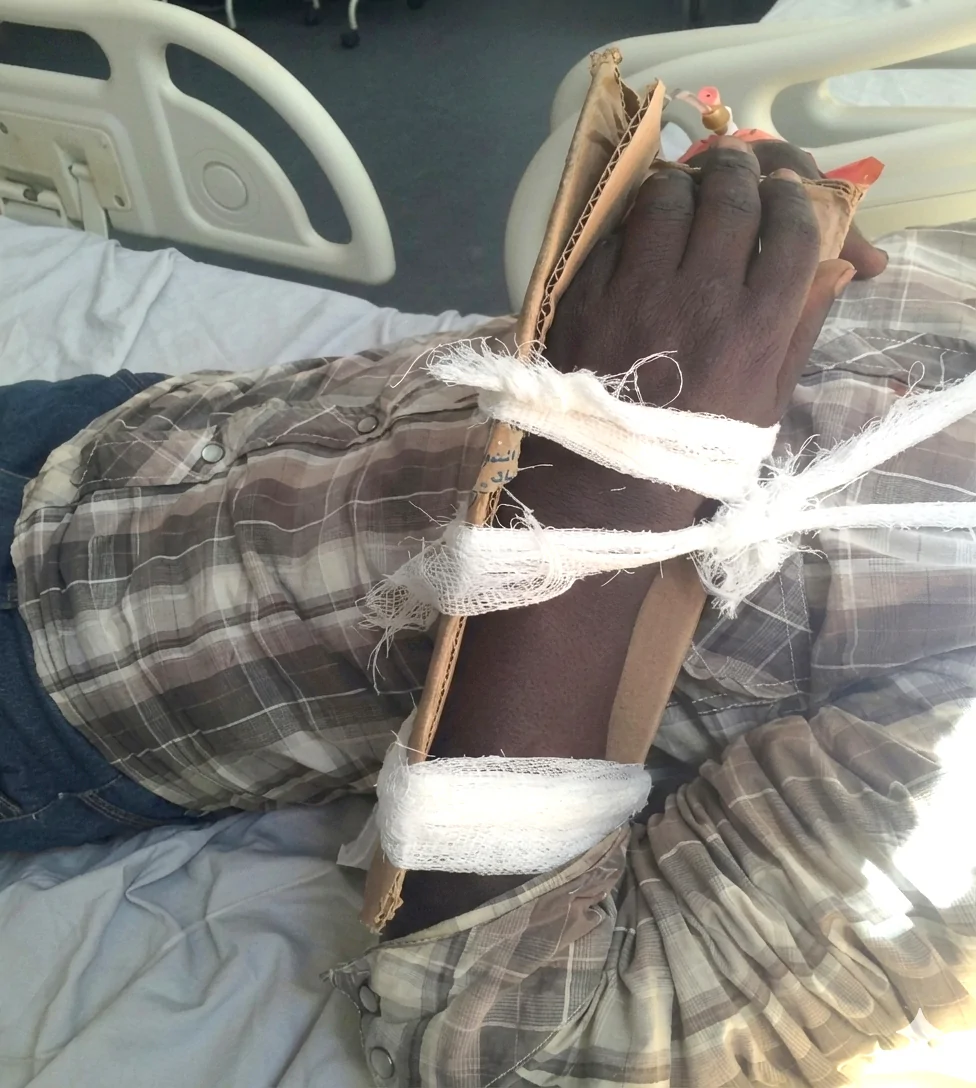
Forearm trauma at health centre (policymaker, Rwanda); IoM priority domain: Effectiveness; Narration: Distal fracture of the right radius. The immobilisation was not done following the standard methods. Even if the health centre used the carton there was inappropriate material to immobilise the fracture. After the X-ray of the wrist, I found that the fracture was not displaced. Therefore, the care received was effective.
